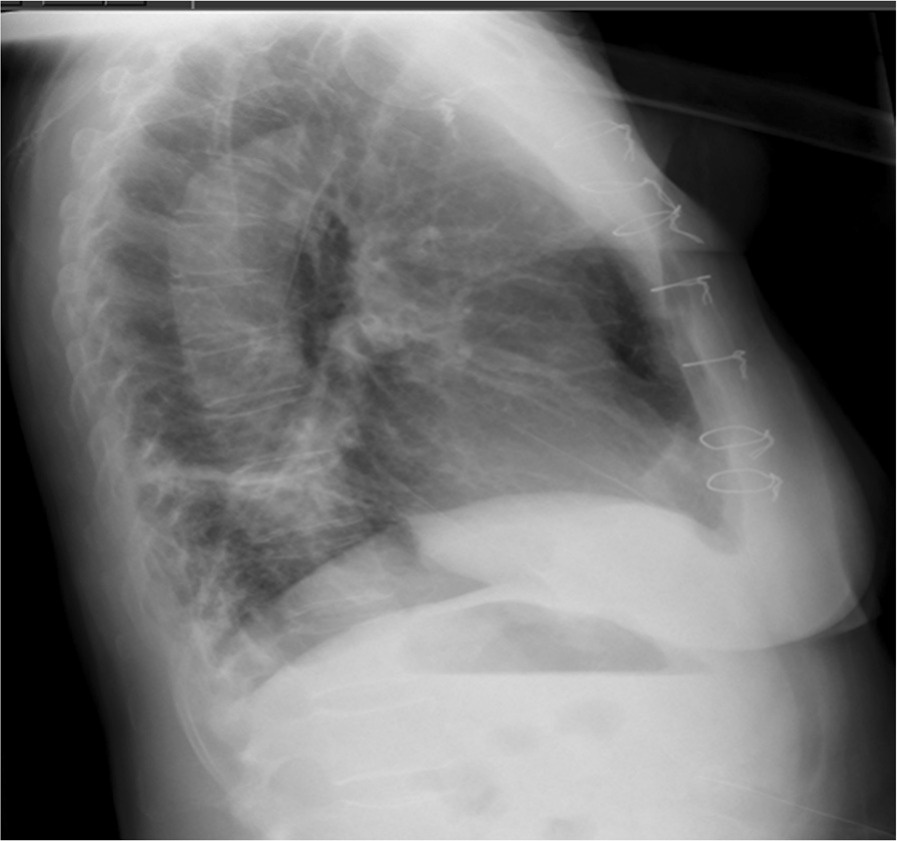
Left lateral chest X-ray showing posterior dislocation of the top sternal wire.
